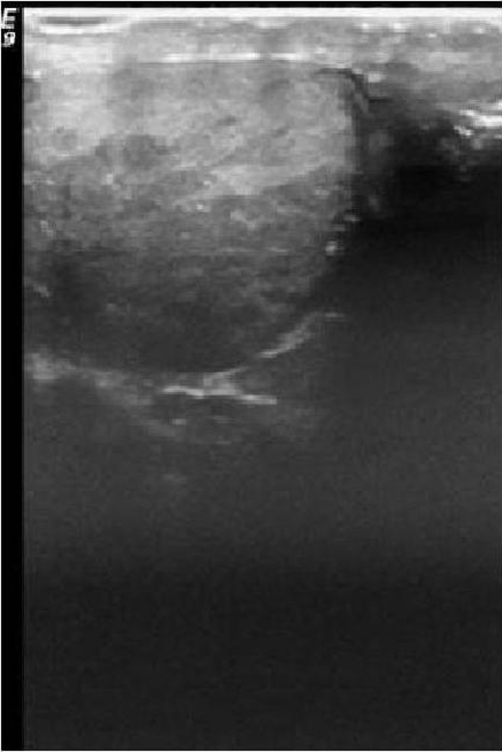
Ultrasound scan of the right testicle showing the non-homogenous abnormality inferiorly (white arrow).
